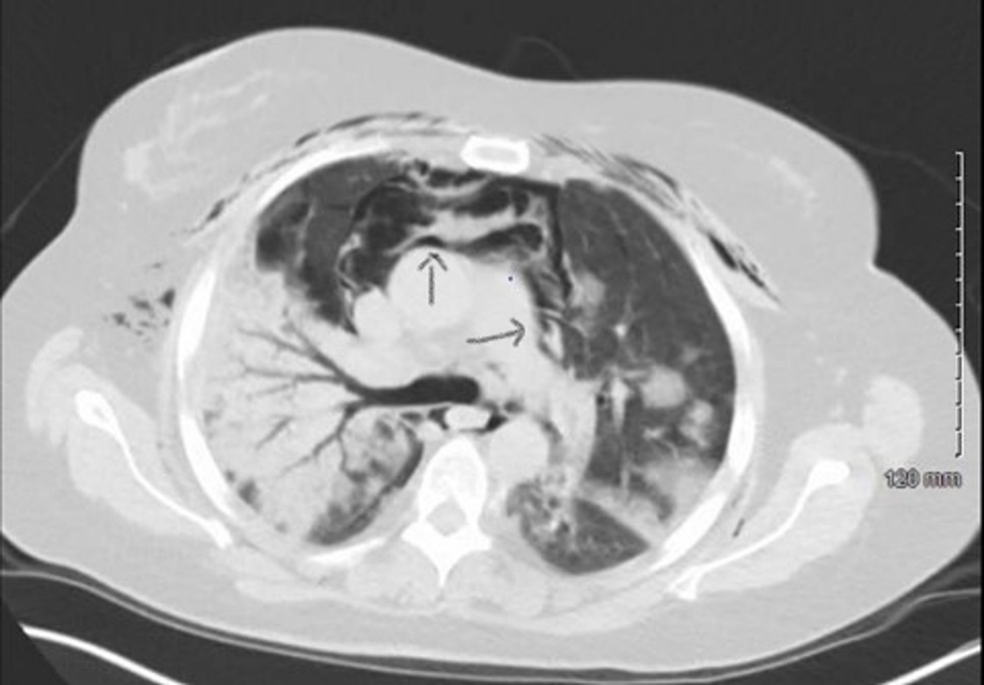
CT chest showing severe diffuse bilateral pneumonia, extensive pneumomediastinum, pneumopericardium, and subcutaneous emphysema
